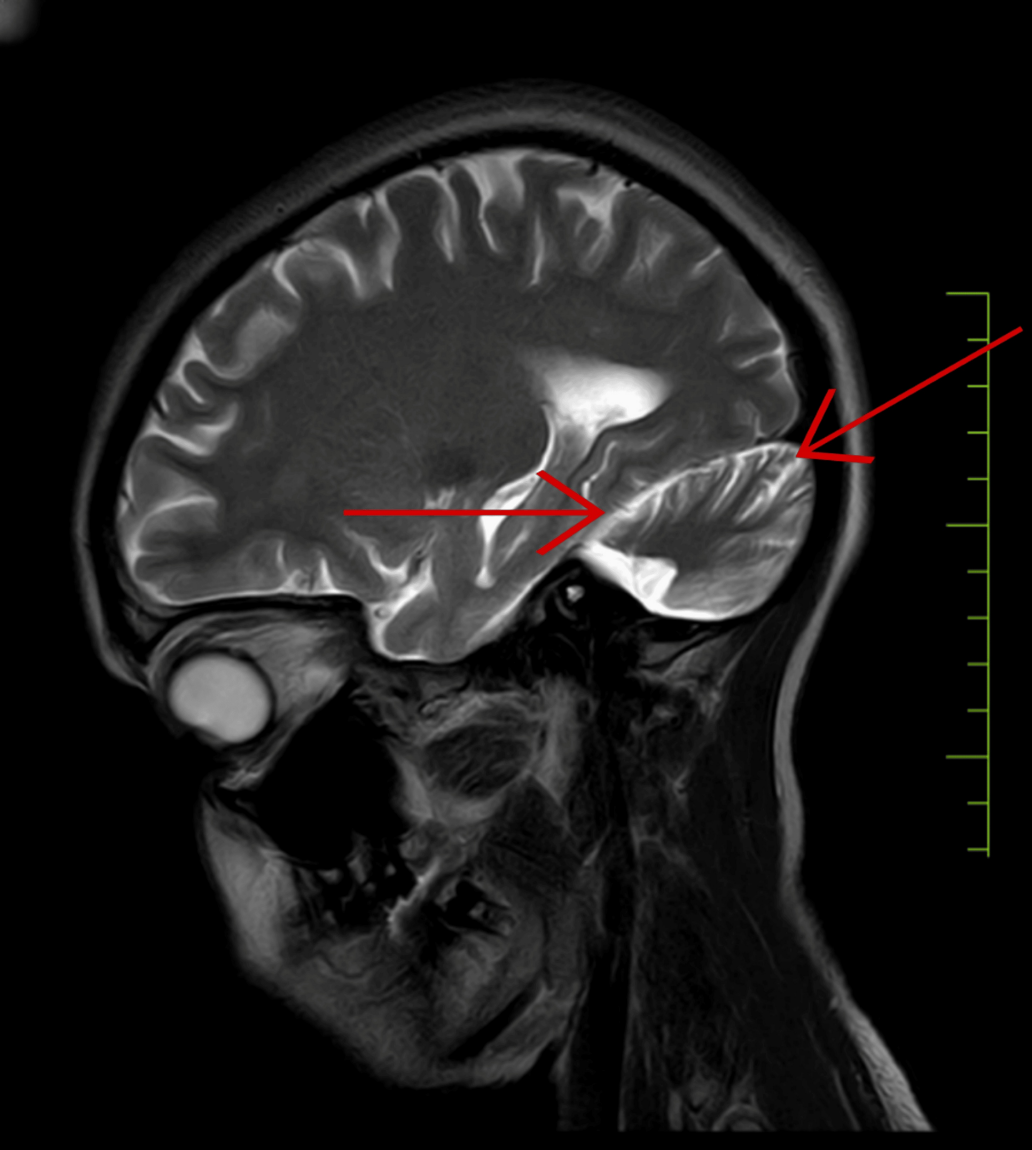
Magnetic resonance imaging (MRI) of the brain showing the atrophy of the cerebellar region
